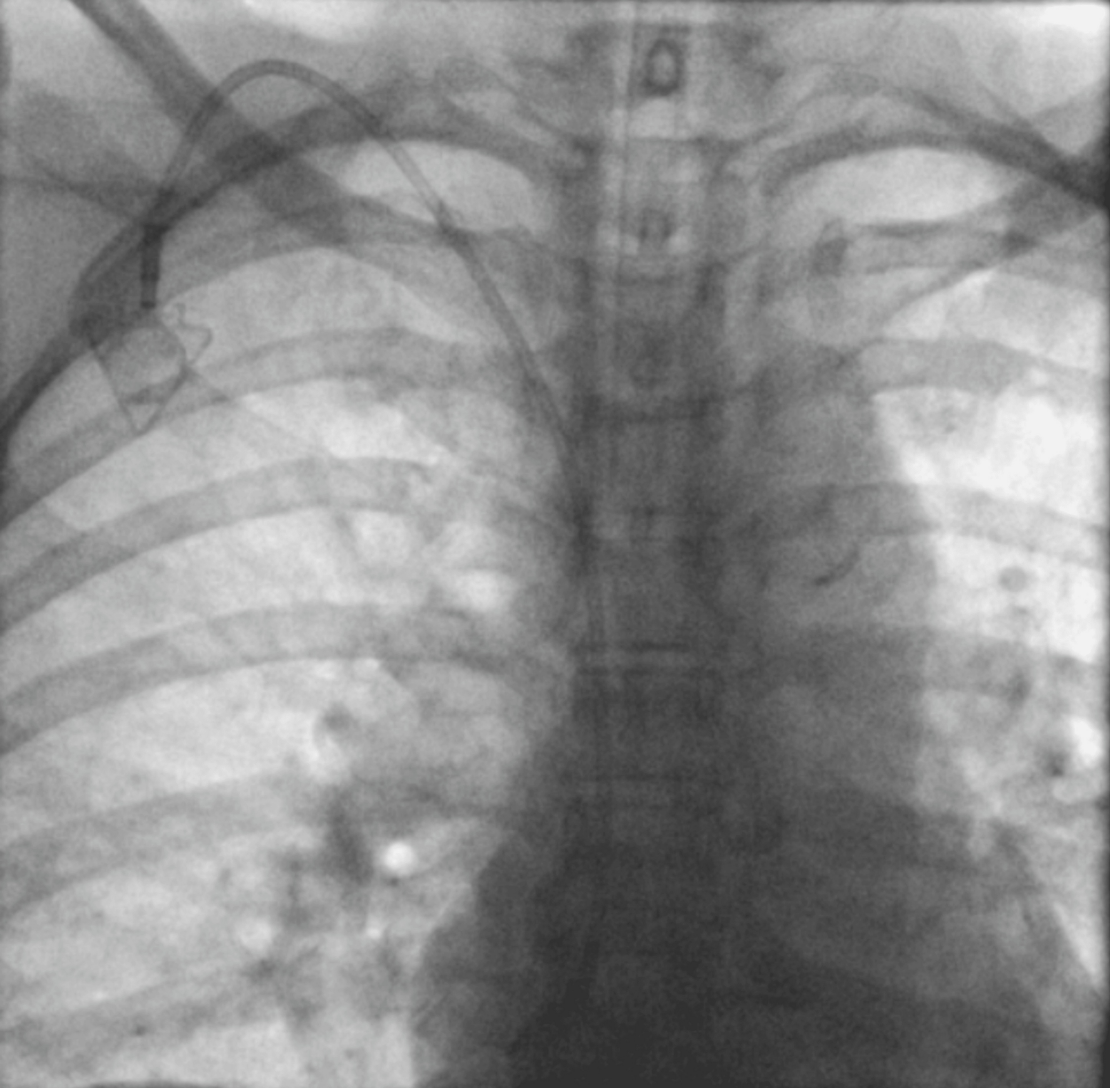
Right internal jugular vein insertion Right-sided implantable port catheter, with the catheter tip positioned superficially within the right atrium. Anteroposterior chest radiograph obtained with a C-arm demonstrating correct catheter placement at the cavoatrial junction.
